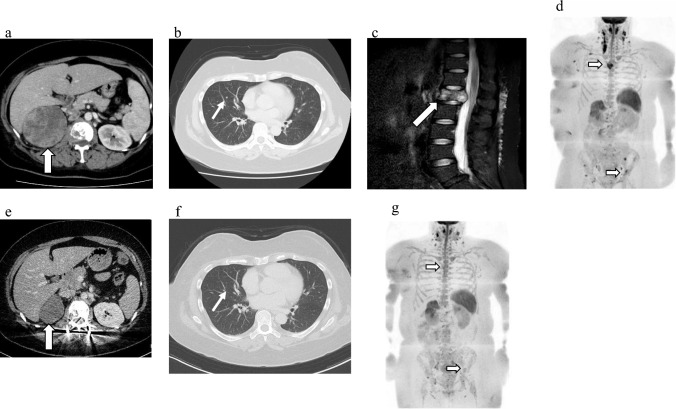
Imaging examinations of case 1 before (a–d) and after (e–g) combined nivolumab and cabozantinib treatment. a Compute tomography (CT) showing primary right renal tumor before combined treatment. b CT showing lung metastatic lesion before combined treatment. c Magnetic resonance imaging showing L1 bone metastatic lesion before combined treatment. d Diffusion-weighted imaging with background suppression (DWIBS) showing metastatic lesions in the sternum and left ilium before combined treatment. e CT showing primary right renal tumor after combined treatment. f CT showing lung metastatic lesion after combined treatment. g DWIBS showing metastatic lesions in the sternum and left ilium after combined treatment
